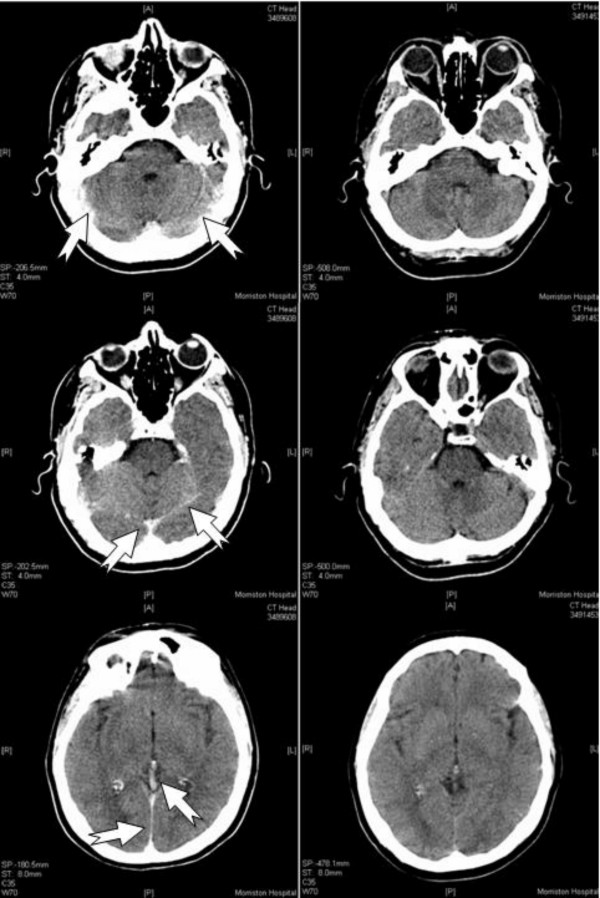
'Unenhanced' computed tomography scan of a brain 4 hours (A) and 24 hours (B) after percutaneous coronary intervention. A) Scan shows high attenuation over the tentorium extending along the falx, over the cerebellar convexities and within the dural sinuses (vein of Galen, straight and transverse sinuses) and smaller veins overlying the tentorium (arrows) with no brain parenchymal or intraventricular enhancement. B) Normal brain scan.
